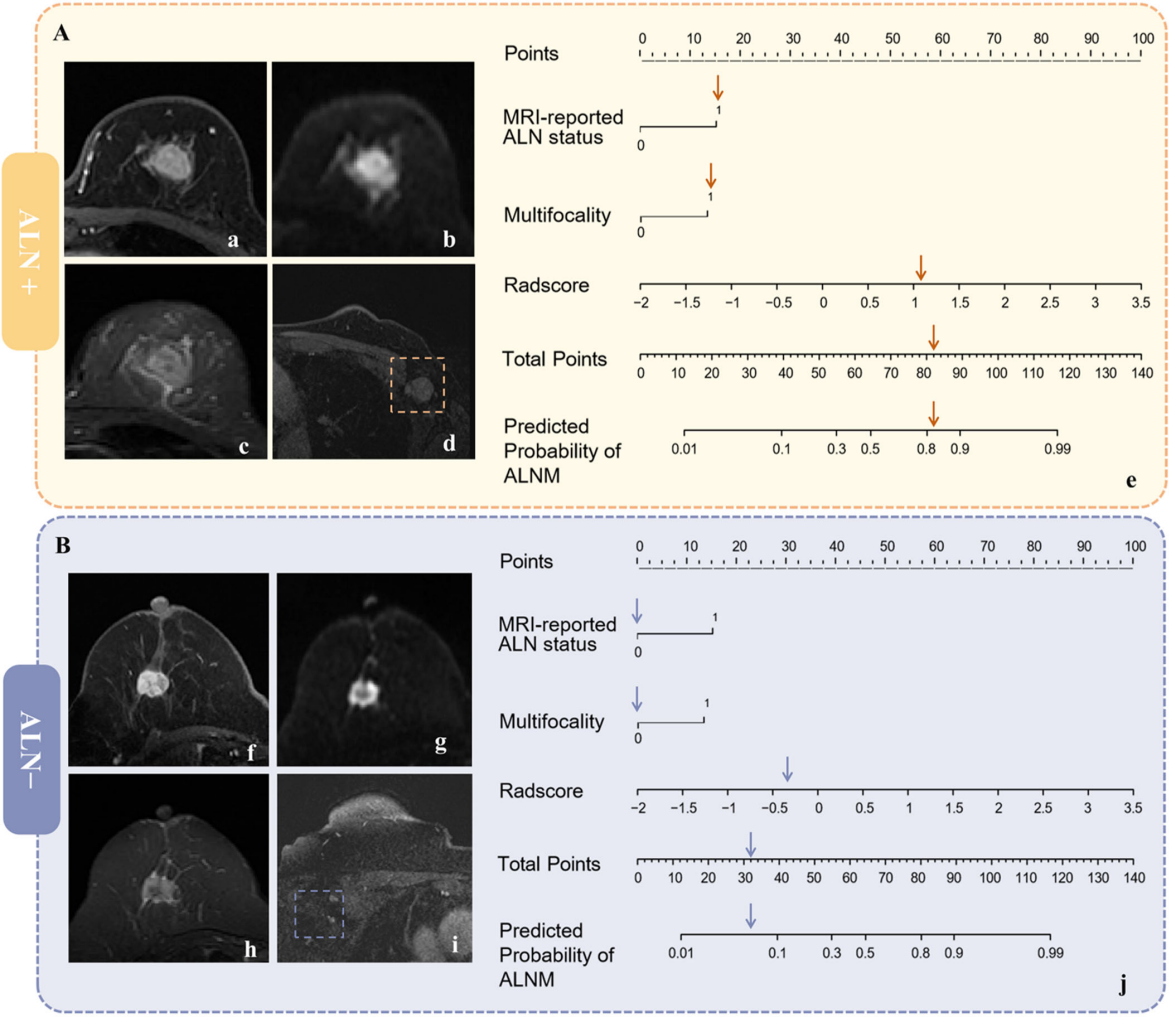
Representative examples of pALN positive and pALN negative. (A) Breast MRI examination of a 60‐year‐old patient with invasive breast cancer, including (a) DCE sequence, (b) DWI sequence, and (c) T2WI sequence. (d) The abnormal ALN reported by MRI was demonstrated on the axial DCE images. (e) The possibility of ALN positivity, assessed by nomogram, could be more than 80%. (B) Breast MRI examination of a 65‐year‐old patient with invasive breast cancer, including (f) DCE sequence, (g) DWI sequence, and (h) T2WI sequence. (i) The normal ALN reported by MRI was demonstrated on the axial DCE images. (j) The possibility of ALN positive assessed by the nomogram could be less than 10%. ALN+, axillary lymph node positive; ALN−, axillary lymph node negative; DCE, dynamic contrast‐enhancement; DWI, diffusion‐weighted imaging; MRI, magnetic resonance imaging; T2WI, T2‐weighted imaging.
